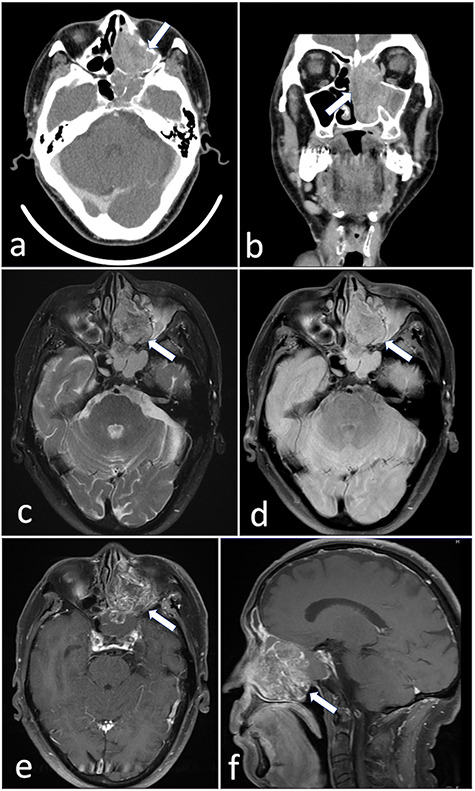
(a and b) CT revealed a tumor 5.5 cm in size in the left nasal cavity (arrow). (c–f) Magnetic resonance imaging revealed a tumor 5.5 × 4 × 3 cm in size occupying the left ethmoidal sinus and left nasal cavity, with invasion of the left orbital cavity, left maxillary sinus and intracranial cavity (arrow).
